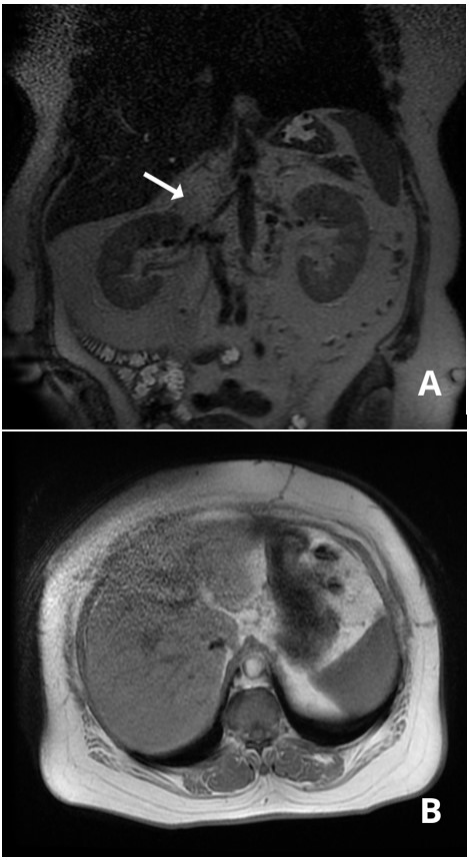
(a) Coronal magnetic resonance image in T2, 17 months after surgery, showing the sectioned segment of the inferior vena cava and absence of local relapse; (b) dual echo axial image showing the retro-hepatic region tumor free after surgery.
